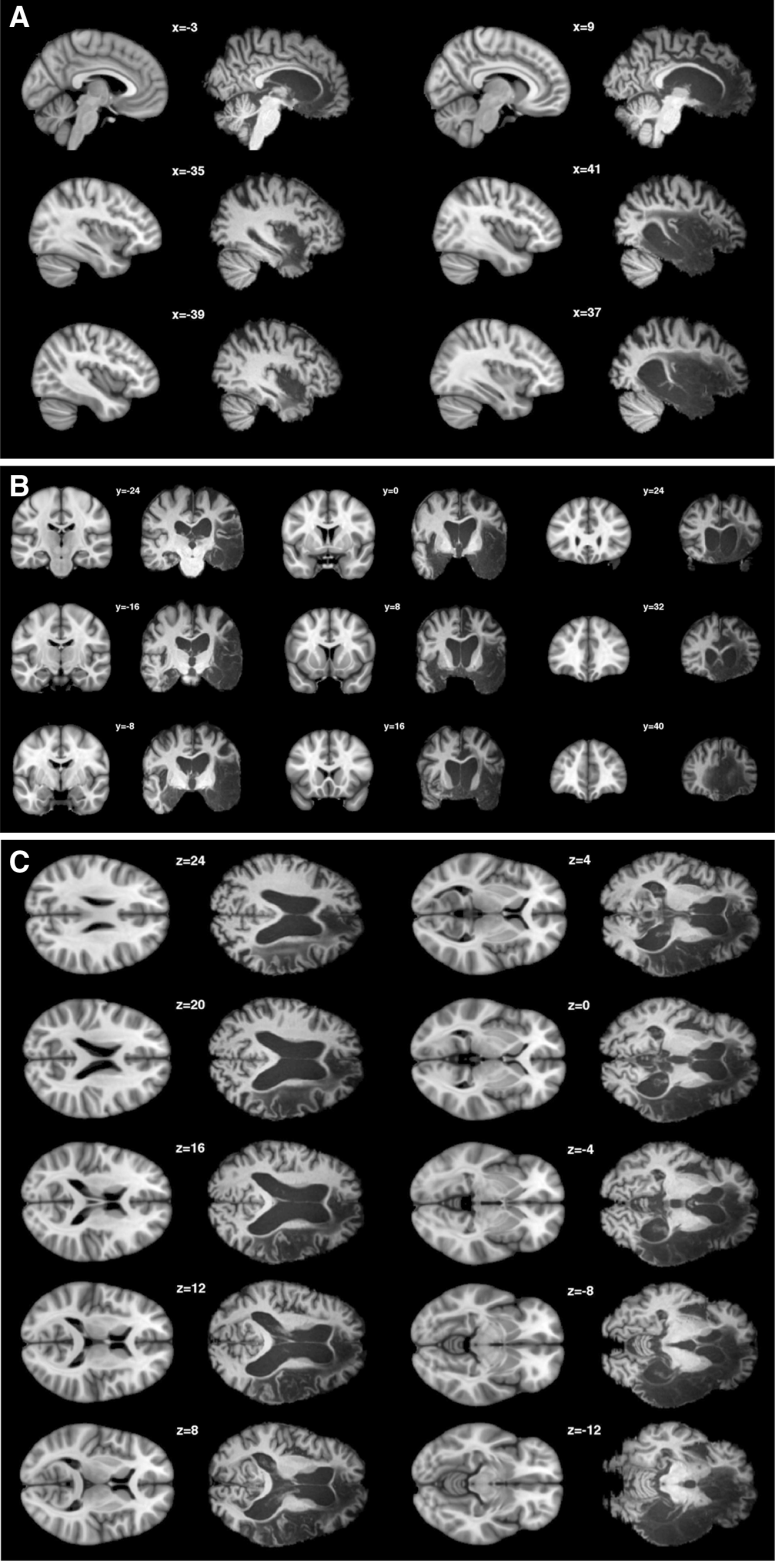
Roger’s brain in comparison to the standard MNI brain. a Sagittal, b coronal, and c axial MRI slices through Roger’s brain placed next to the same slice from the standard MNI brain
